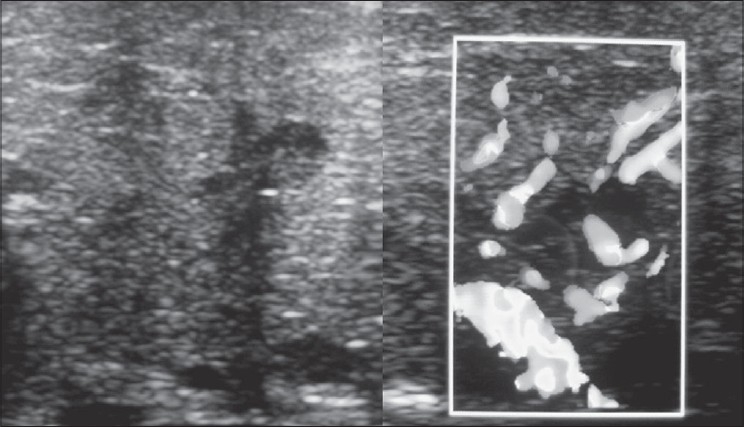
Ultrasound of the parotid gland showing diffuse swollen gland with hypoechoic areas (left) and increased vascularity (right)
